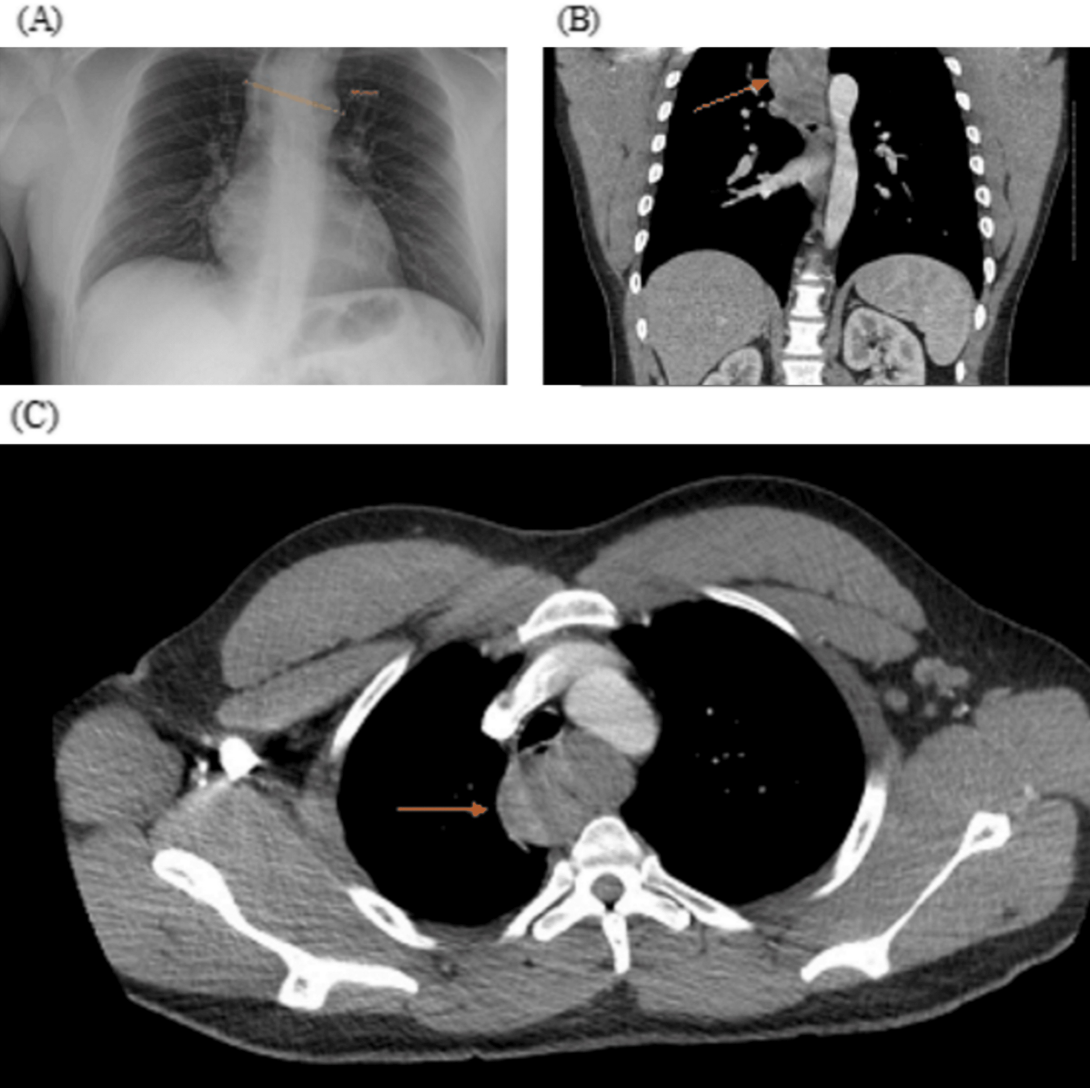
Preoperative imagining (A) Upright chest X-ray with the mediastinal mass measuring 85 mm. (B) Coronal and (C) Axial CT scan demonstrating esophageal soft tissue mass (arrows indicate the mass)
